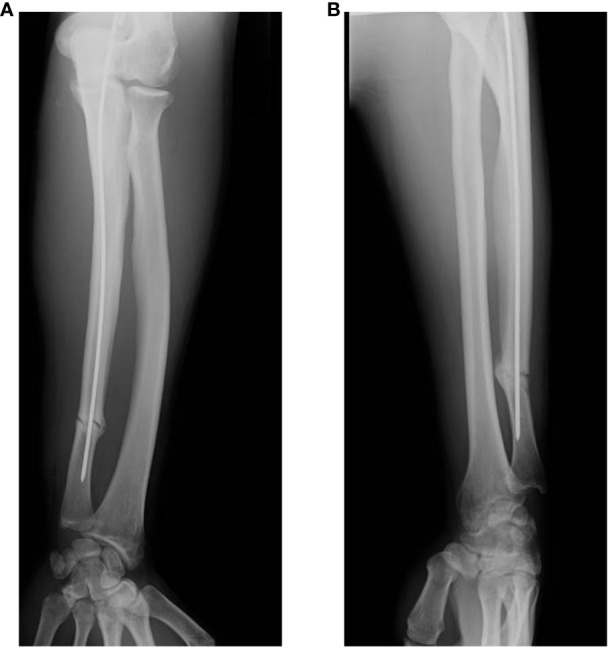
Radiographs at 3 months after surgery. Fracture consolidation is still not achieved as shown by anterior–posterior (A) and lateral (B) X-rays of the left ulna.
